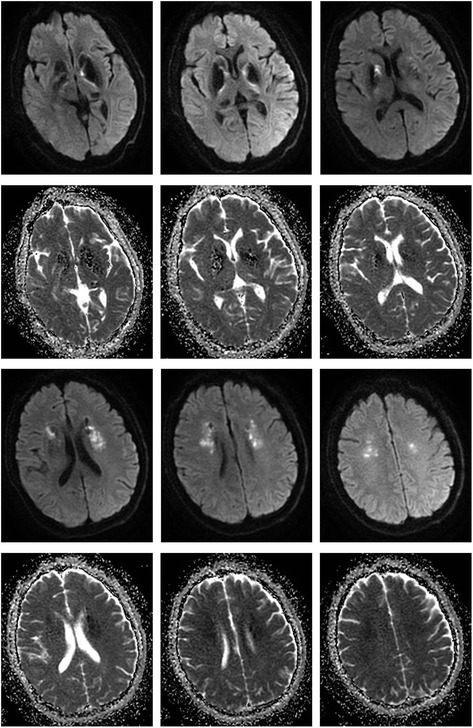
Brain magnetic resonance imaging (MRI). Hyperintense signal in diffusion weighted imaging (DWI) and hypointense signal in apparent diffusion coefficient (ADC) in bilateral basal ganglia and periventricular regions
